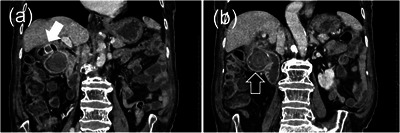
Computed tomography showing calcified stones (white arrow) and gas in the gallbladder with a cholecystoduodenal fistula (a) and a stone (black arrow) in the duodenal bulb (b).
